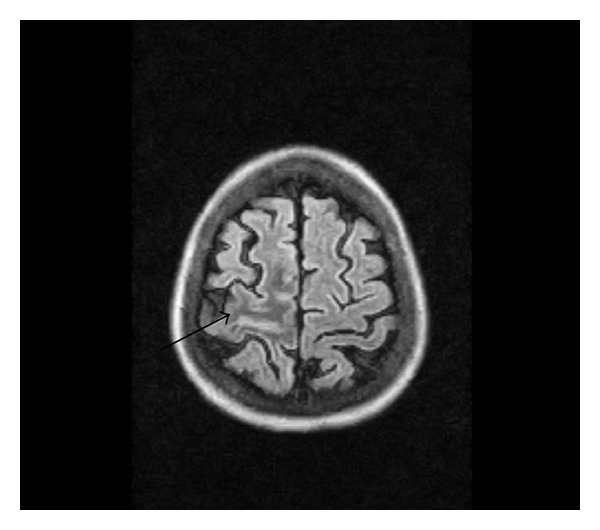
Axial T2 FLAIR image shows subcortical T2 hypointensity in right pre- and postcentral white matter.
